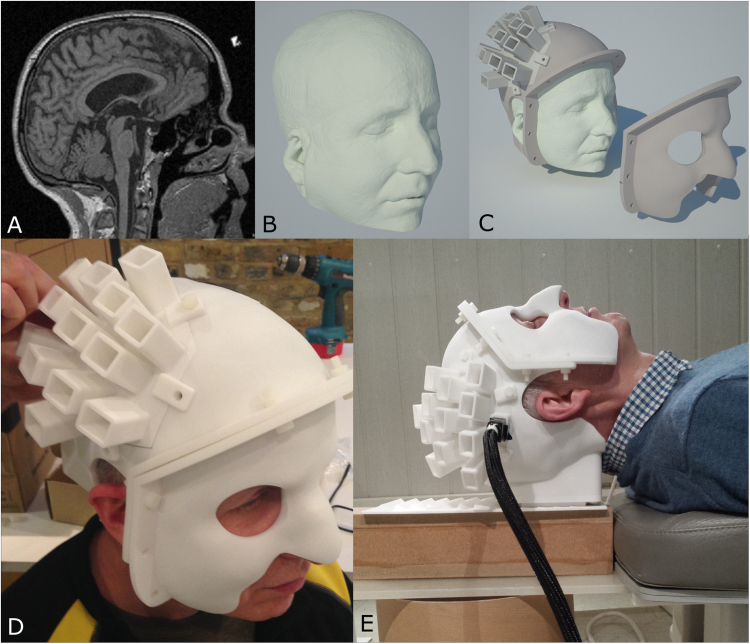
Head-cast design and fabrication: A) Single sagittal slice from the anatomical MRI. B) Outer head surface extracted from MRI. C) CAD model of the head-cast with slots designed to house the OPM sensors over sensorimotor cortex. Slot positions are based on a-priori prediction of the spatial topography of scalp field pattern, derived from previous SQUID measurements in the same subject. D) 3D-printed head-cast on subject. E) Subject in situ with the OPM attached. Note that the head-cast is not only fixed rigidly to the subject's scalp, but is also fixed relative to the MSR, thus eliminating any sensor motion relative to the subject, and subject motion relative to the MSR.
